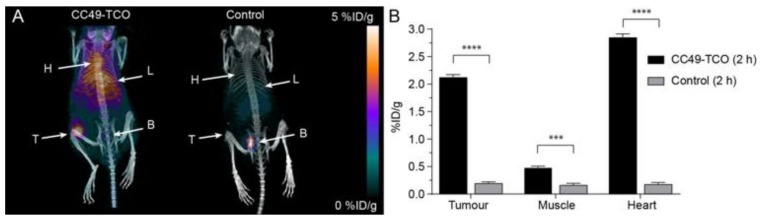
Pretargeted PET/CT imaging of [^68^Ga]3 targeting CC49-TCO (5), which was administered 24 h prior to the administration of [^68^Ga]3. (A) Respective PET/CT images after 2 h. (B) Image-derived quantitative analysis of tissue uptake. * *p* < 0.001 and  *p* < 0.0001.
